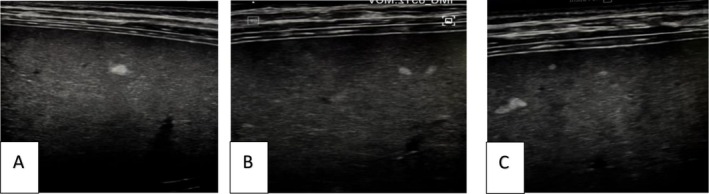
Abdominal ultrasound of the liver depicted hepatomegaly with a normal echo pattern. Several small echogenic nodules were seen during the examination of the liver with a linear probe.
